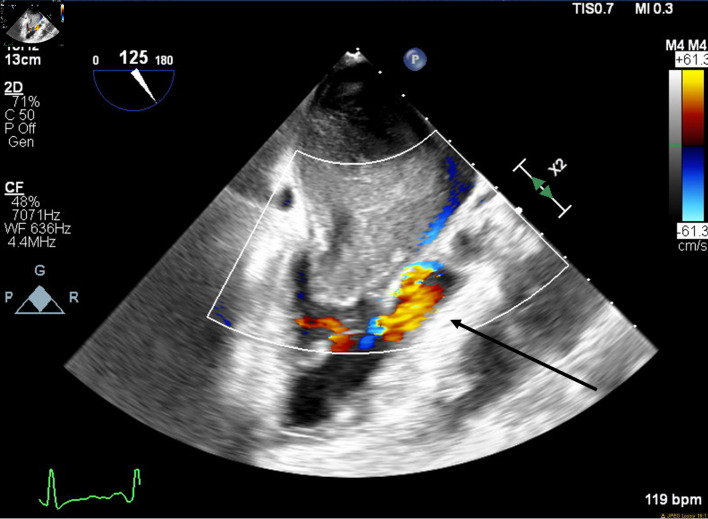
Mid-esophageal aortic long-axis view with color flow Doppler demonstrating turbulent flow through left ventricular outflow tract (LVOT) during early systole. The arrow indicates turbulent flow through the LVOT.
